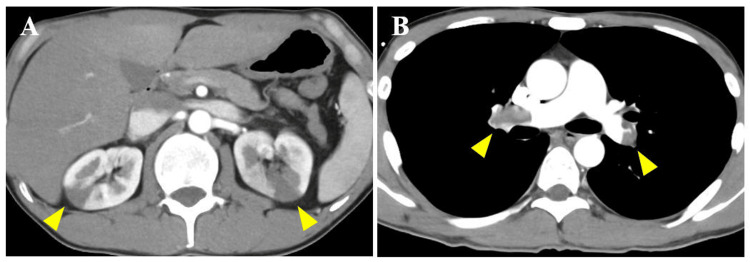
Contrast-enhanced computed tomography and pulmonary CT angiography (A) Abdominal contrast-enhanced computed tomography (CT) revealed bilateral renal infarctions (yellow arrowhead). (B) Pulmonary CT angiography revealed bilateral pulmonary embolisms (yellow arrowhead).
